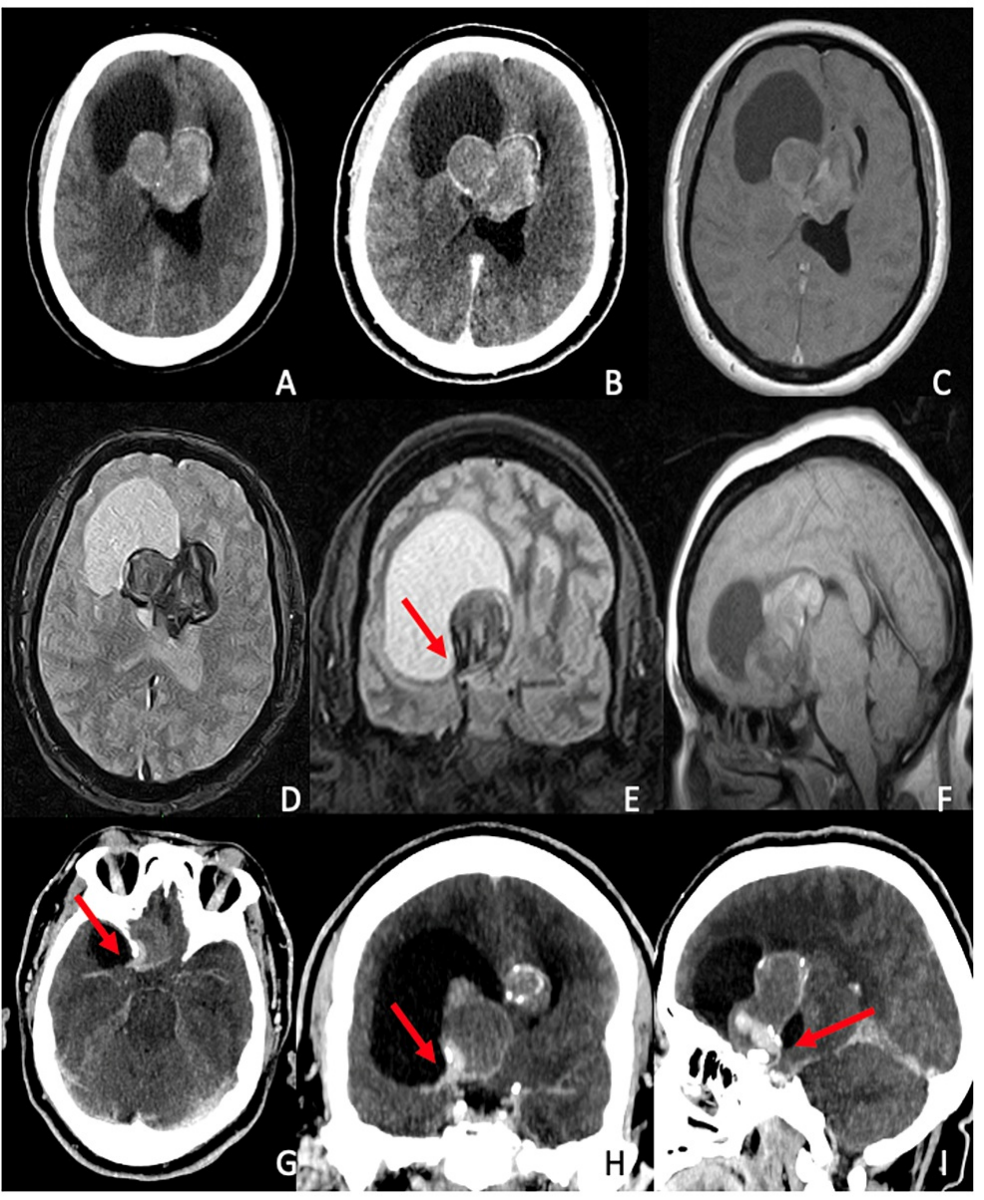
Preoperative neuroimaging assessments Axial section contrast-enhanced CT scan showed a midline mass with peripheral calcifications (A) and heterogeneous contrast enhancement (B). The right frontal cystic lesion appears more hyperintense than the CSF in contrast-enhanced MRI sequences. SWI reconstructions evidenced flow voids (D, E) and heterogenous contrast enhancement is seen on sagittal T1 (F). Prior radiology report suggested craniopharyngioma. A contrast-enhanced CT scan adjusted for arterial vessels evidenced a hyperdense pattern within the tumoral wall, suggesting luminal patency (red arrows) from the right ICA bifurcation CT: computed tomography; CSF: cerebrospinal fluid; MRI: magnetic resonance imaging; SWI: susceptibility-weighted imaging; ICA: internal carotid artery
